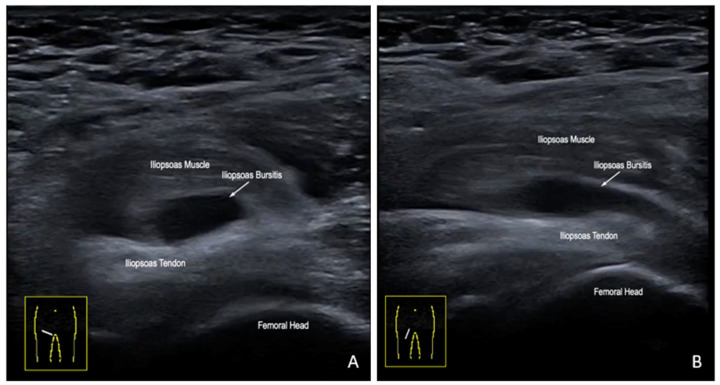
Overuse iliopsoas bursitis. Ultrasound images obtained over the femoral head in the transverse (A) and longitudinal sagittal (B) planes demonstrate a well-defined anechoic fluid distention of the iliopsoas bursa, located deep to the muscular portion of the iliopsoas, consistent with bursitis. Mild thickening of the adjacent iliopsoas tendon suggests associated early tendinopathic changes.
